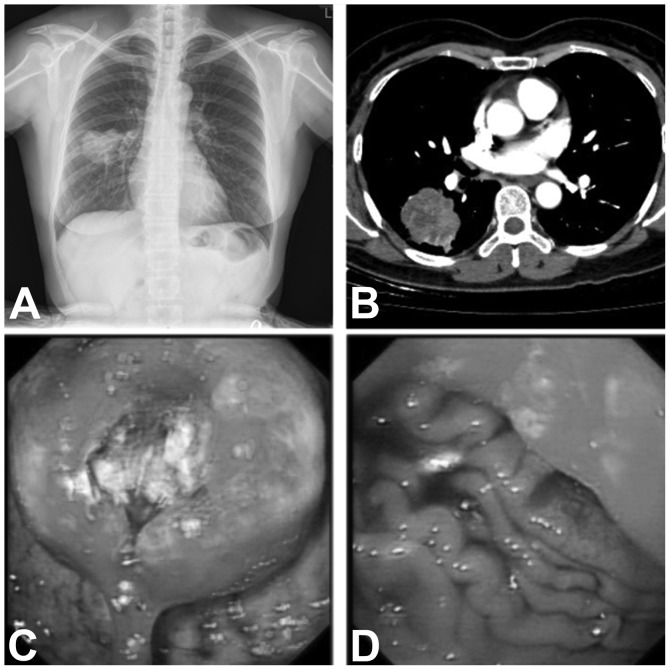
(A) Chest X-ray revealing an abnormal mass shadow in the right lower lobe. (B) Computed tomography scan of the chest demonstrating an irregular mass measuring 39×48 mm in size, with a scallop-shaped contour and focal enhancement. (C and D) Gastroscopy images revealing a mass, ~4×4 cm in size, in the fundus of the stomach, invading the cardia, with a deep ulcer in the center.
